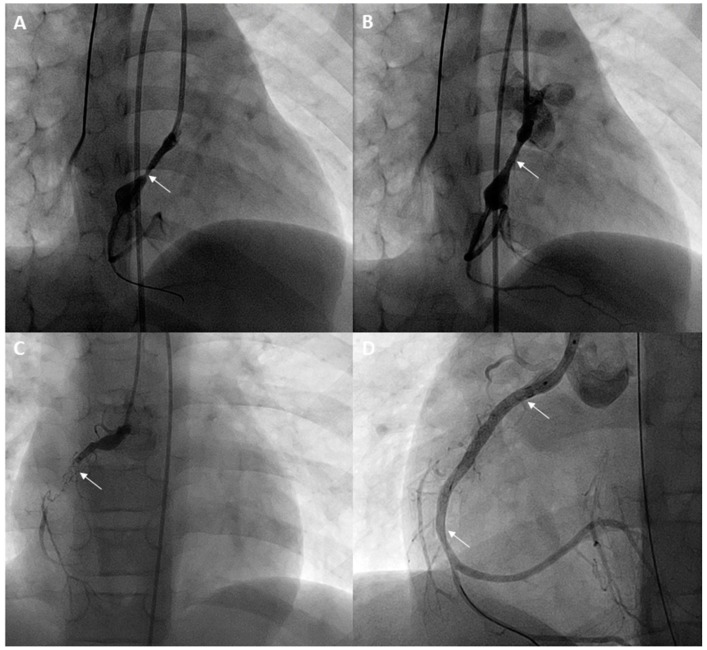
A 7-year-old with a history of Kawasaki disease and giant coronary artery aneurysms of the left main coronary artery and right coronary artery underwent a cardiac catheterization. Fractional flow reserve with adenosine in the right coronary artery (RCA) was 0.74. Stenosis proximal to the aneurysm in the RCA is seen (arrow) (A). After placement of a drug-eluting stent, the stenosis has resolved (arrow) (B). Two years later, a cardiac MRI showed an inducible perfusion defect in the inferior septum and inferior wall. Angiograms in the RCA showed complete occlusion of the RCA stent (arrow) (C). After recanalization and angioplasty, an overlapping stent was placed extending to the distal RCA (lower inferior arrow) (D) and an additional proximal stent was also placed (superior arrow) (D), reestablishing flow in the RCA.
